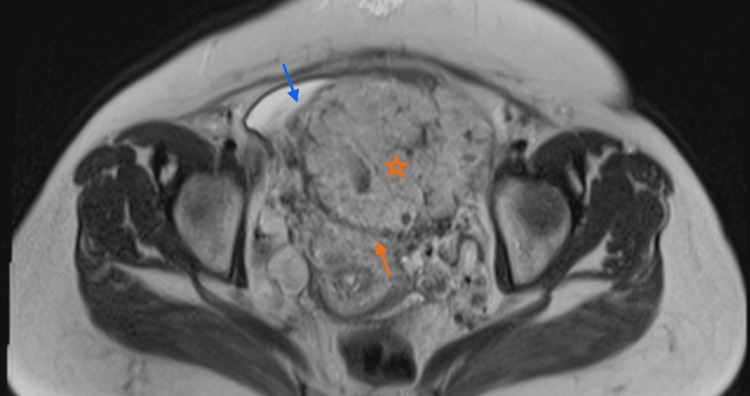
Magnetic resonance imaging in T2 sequence without fat saturation in the axial plane. Placenta with a heterogeneous appearance (star) with a focal exophytic mass at the level of the uterovesical junction, extending towards the bladder (blue arrow). In the posterior segment, the preserved hypointense retroplacental line is observed (orange arrow).
